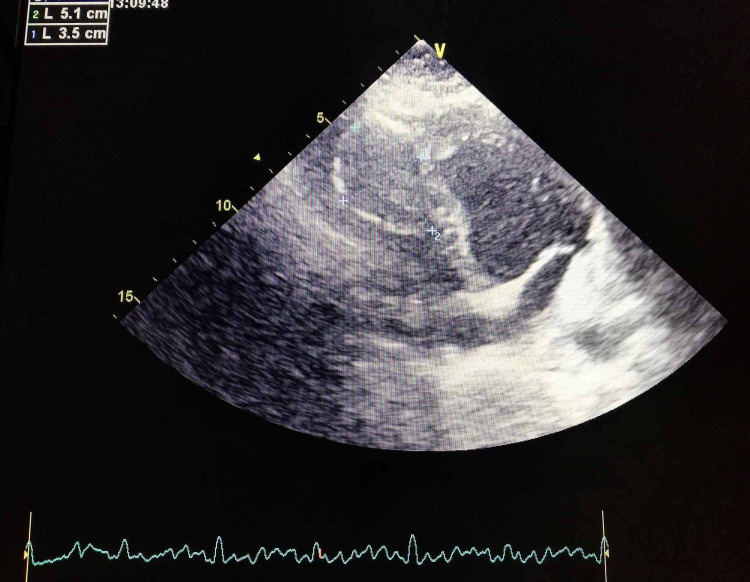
Transthoracic echocardiography showing the hydatid cyst in the interventricular septum.
